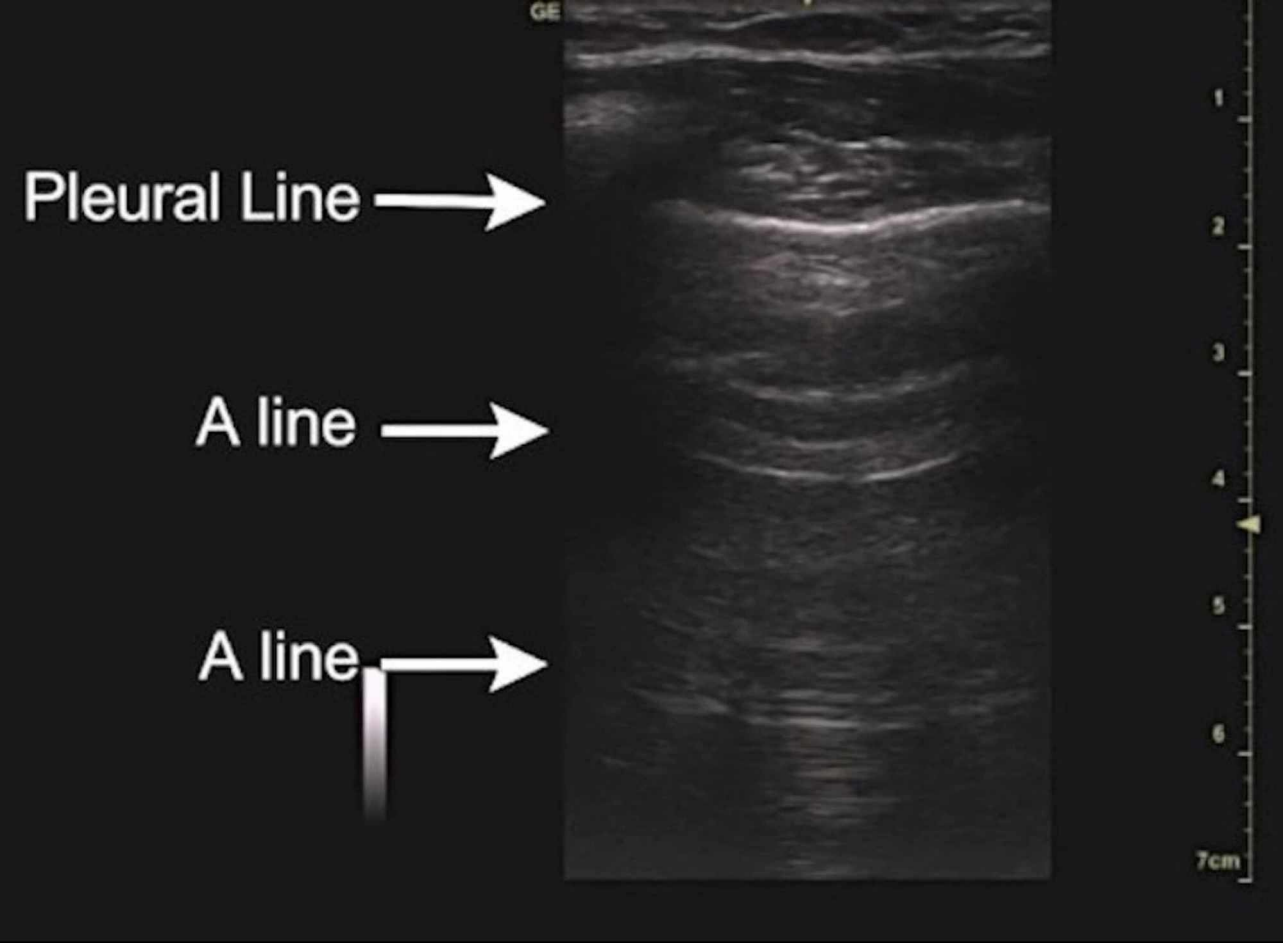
A lines are an important component of the normal lung ultrasound profile
